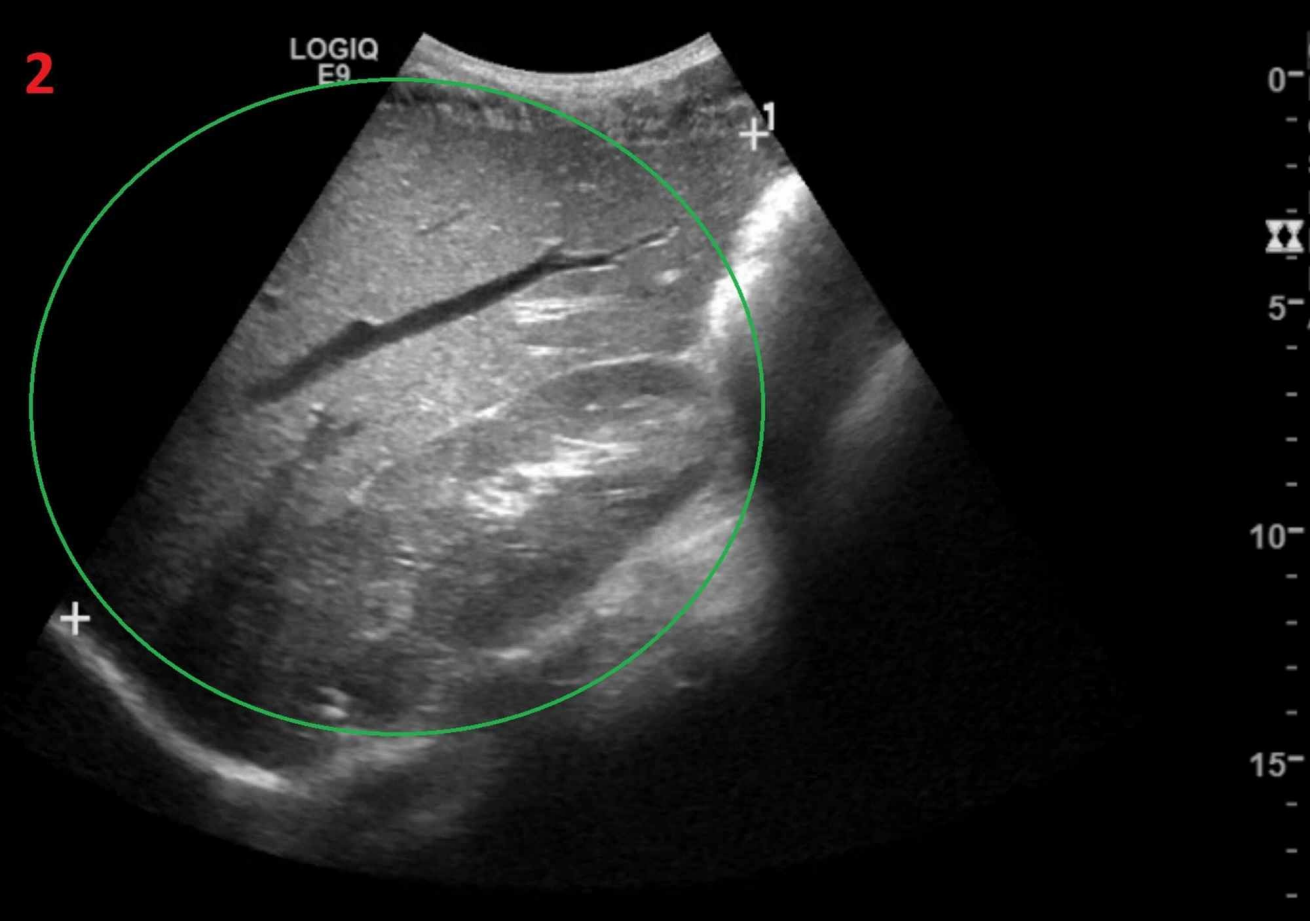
Ultrasound of the liver Enlarged liver seen (green circle)
